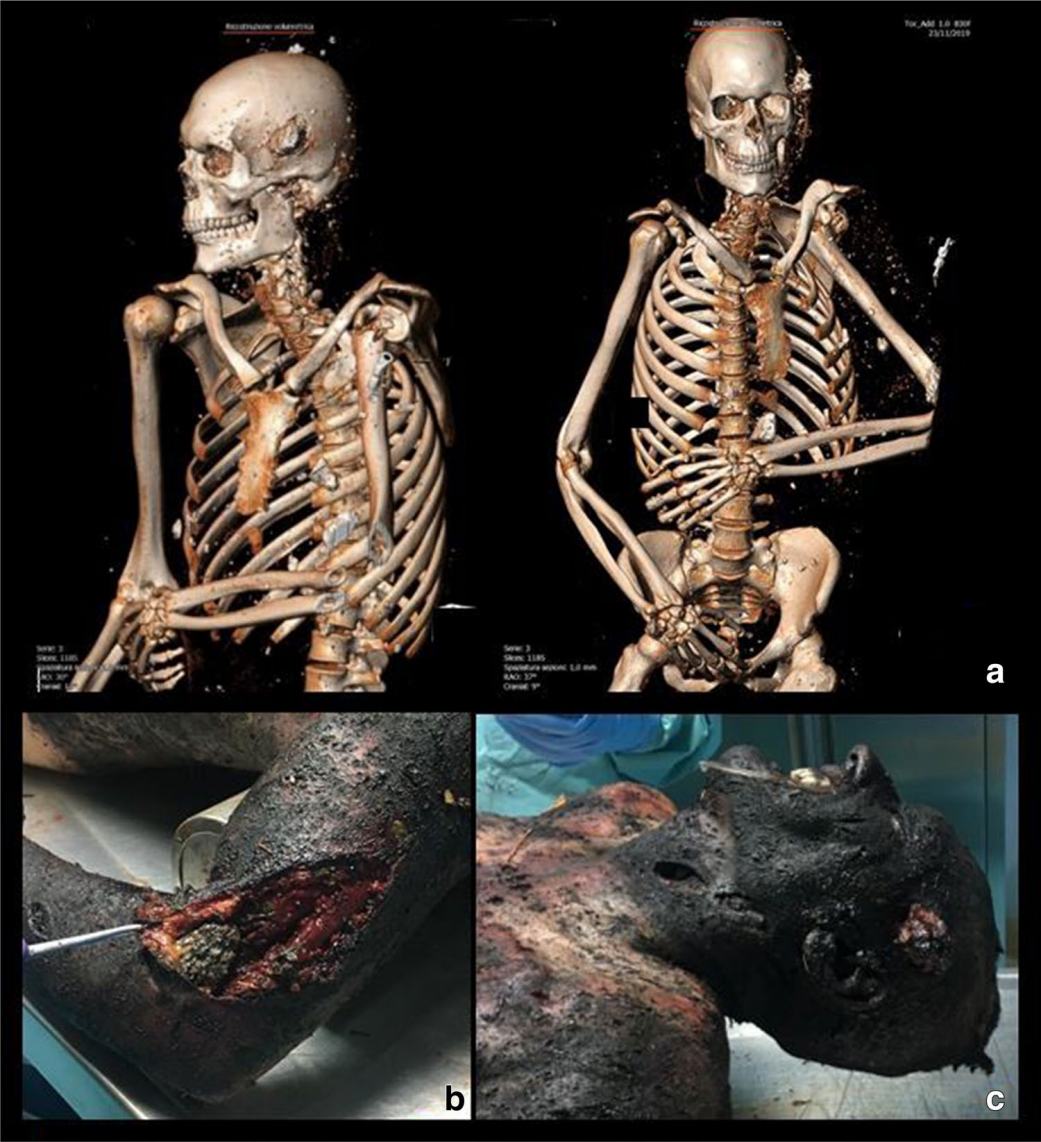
Subject no. 2: 3D CT scan (a) and details of the lesions detected on external inspection (b and c)
